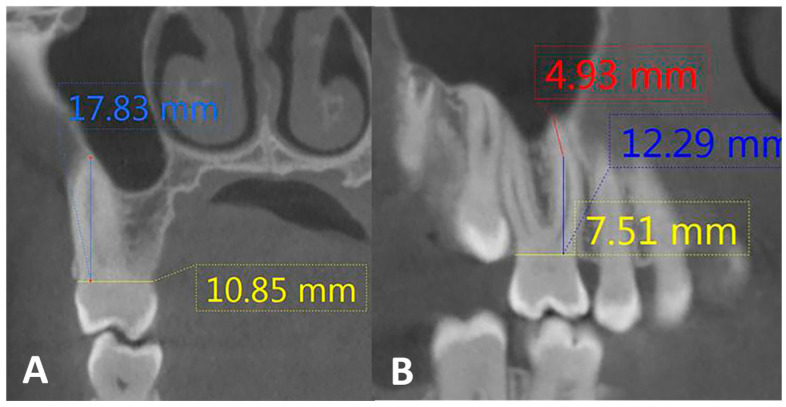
CBCT reconstructions of MB root in maxillary first molar tooth. Root length measurement in (A) coronal section, (B) curved MB root.
